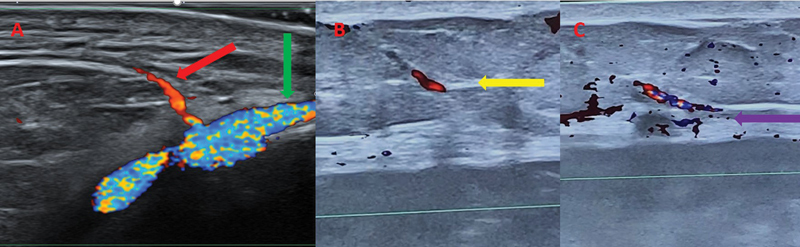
Preoperative color Doppler ultrasonography of the recipient site and anterolateral thigh flap. (
